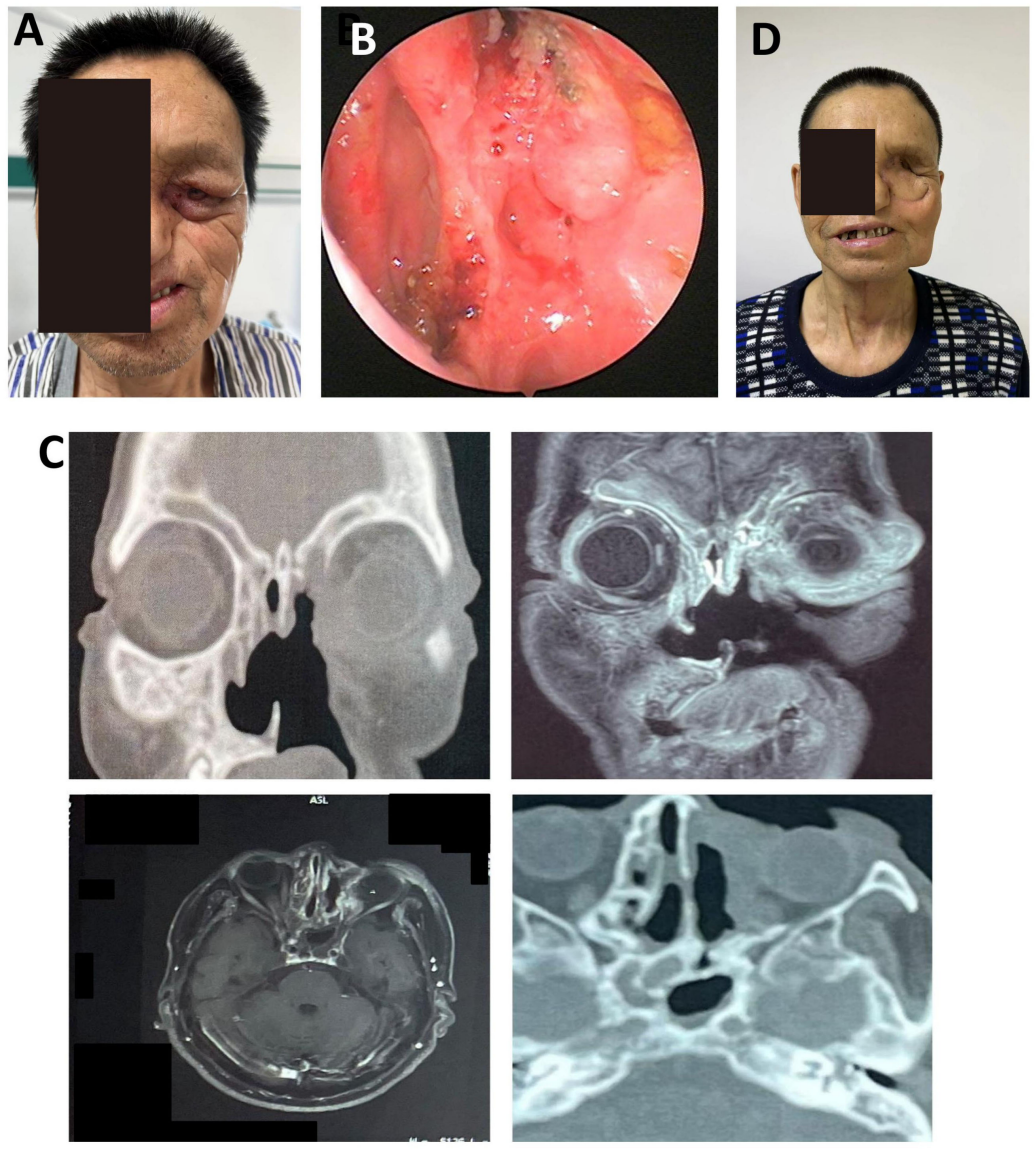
Postoperative orbital-maxillofacial changes and imaging findings in a patient with recurrent maxillary sinus carcinoma. (A) Preoperative facial appearance showing narrowed palpebral fissure, swollen and adherent eyelids, and a phthisical globe. Maximal interincisal opening approximately 4.5 cm. (B) Crusted debris observed in the left nasal cavity and irregular intraorbital mass. (C) Axial enhanced MRI (3.0T) and 3D-CT (64-slice) images showing orbital and sinonasal involvement. (D) Facial appearance at the 4-month follow-up demonstrated stable mucosa, adequate flap perfusion, and a well-healed surgical site.
